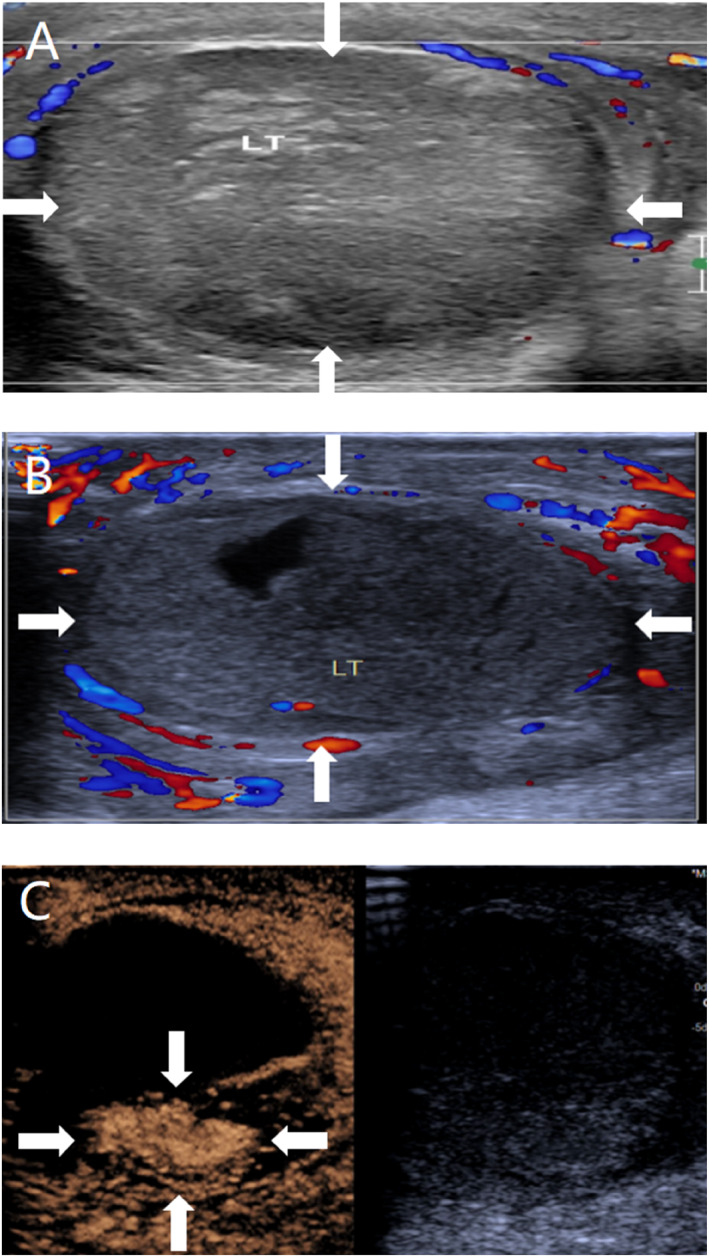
An 11.2‐year‐old male, pathologically diagnosed with necrosis of the left testis (LT) after torsion and treated with left testicular repositioning. (A) Before surgery, conventional ultrasound revealed that the axial position of the left testis had changed, with heterogeneous parenchymal echogenicity, and no blood flow signal was observed in the left testis by color Doppler flow imaging (CDFI; arrowheads). (B) After repositioning, conventional ultrasound revealed that the left testis had inhomogeneous echogenicity, with echogenic areas seen in the left testis, and no blood flow signal was observed in the center of the testis by CDFI (arrows). (C) After repositioning, most of the affected area did not experience contrast infusion, but enhancement was observed in a small area (arrows).
